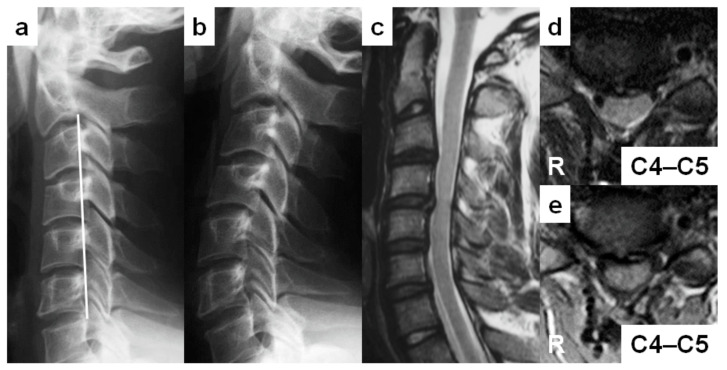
Report of a 24-year-old man with cervical spondylotic myelopathy (case no. 3). (a,b) Lateral cervical spine radiographs in neutral (a) and full-extension (b) positions demonstrating the “reverse-sigmoid” sagittal cervical alignment and posterior vertebral slip at C4. (c) A sagittal T2-weighted magnetic resonance image (MRI) demonstrating “degenerative” discs from C3–C4 to C6–C7, spinal cord compression at C4–C5, and no intramedullary increased signal intensity lesions. (d) An axial T2-weighted MRI demonstrating the narrowed cervical spinal canal without any intramedullary increased signal intensity areas at C4–C5. (e) An axial T1-weighted MRI demonstrating the “ovoid” spinal deformity at C4–C5.
